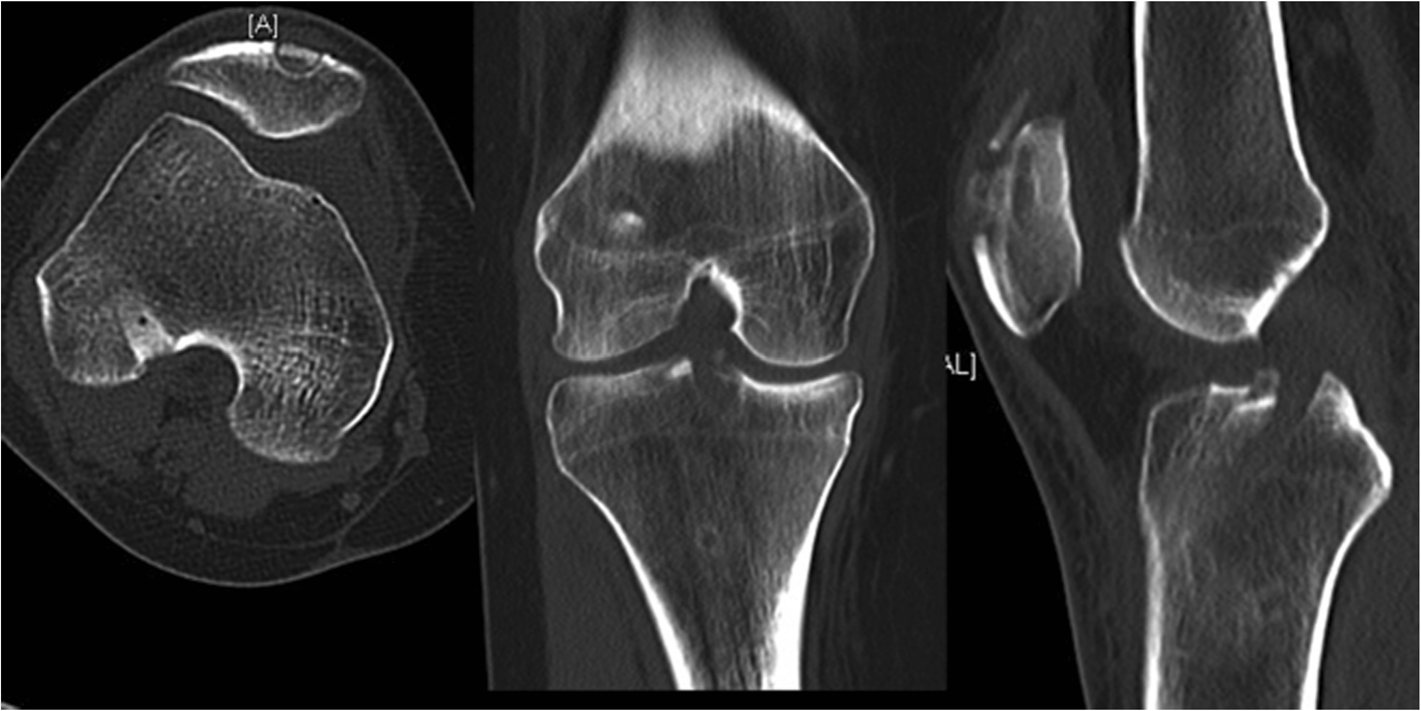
CT scan of the right knee 6 weeks postoperatively.
